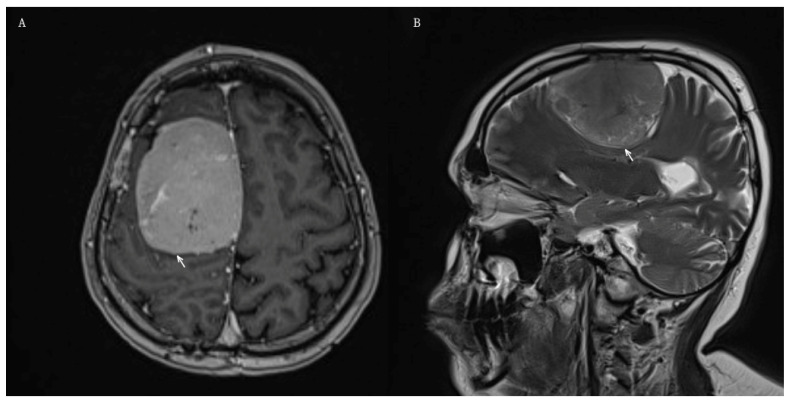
Preoperative MRI of Patient 4 demonstrating a RIM located in the right cerebral convexity. White arrows indicate the tumors. (A) Axial T1-weighted post-contrast image showing homogeneous enhancement of the tumor. (B) Sagittal T2-weighted image.
